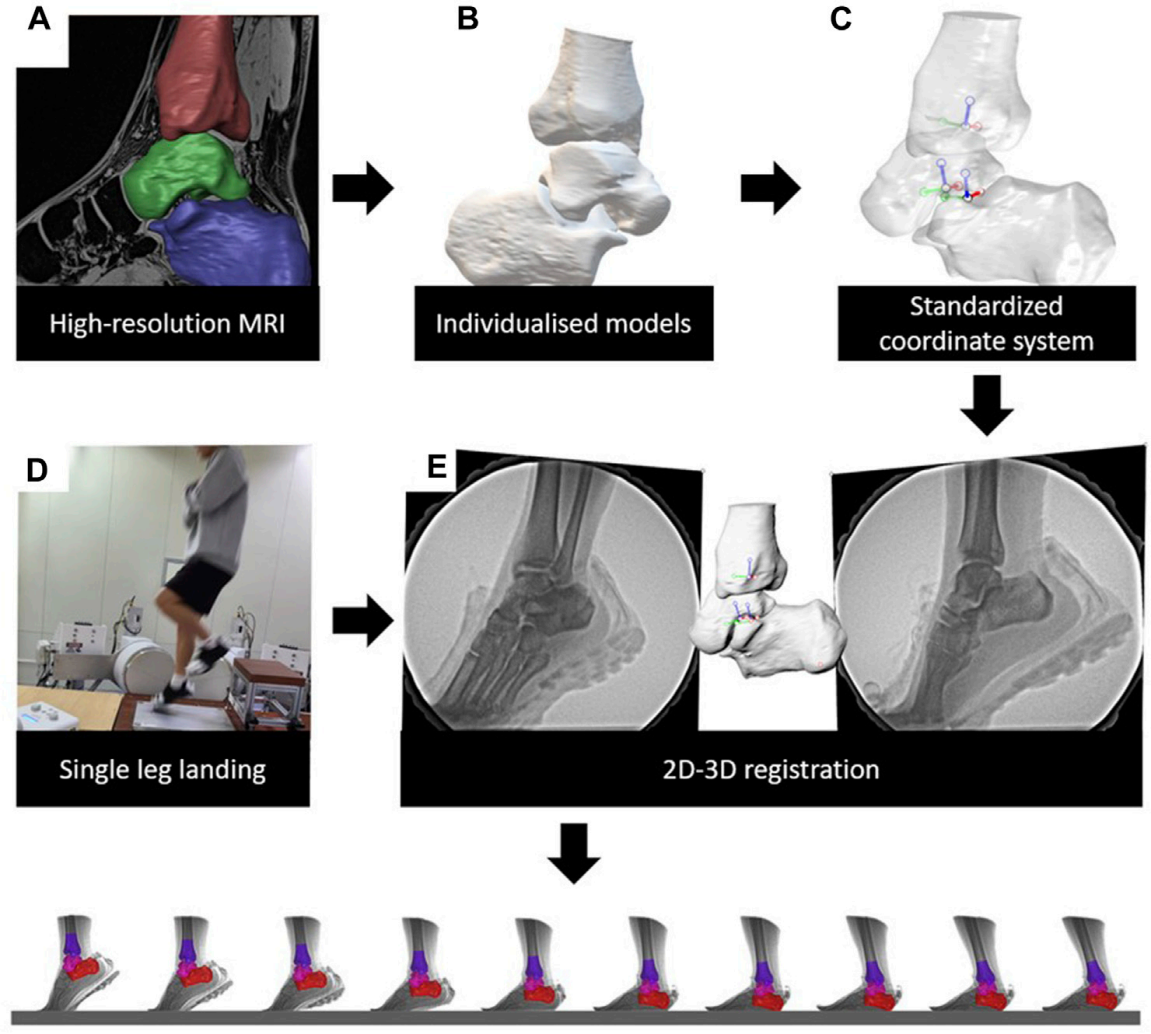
Data collection and process of DFIS. (A) High resolution MRIs were collected. (B) Acquisition of individualized 3D models. (C) Creation of coordinate systems for each model. (D) Acquisition of high-speed dual fluoroscopic imaging system data. (E) Combine MRI and DFIS data to acquire the kinematics.
